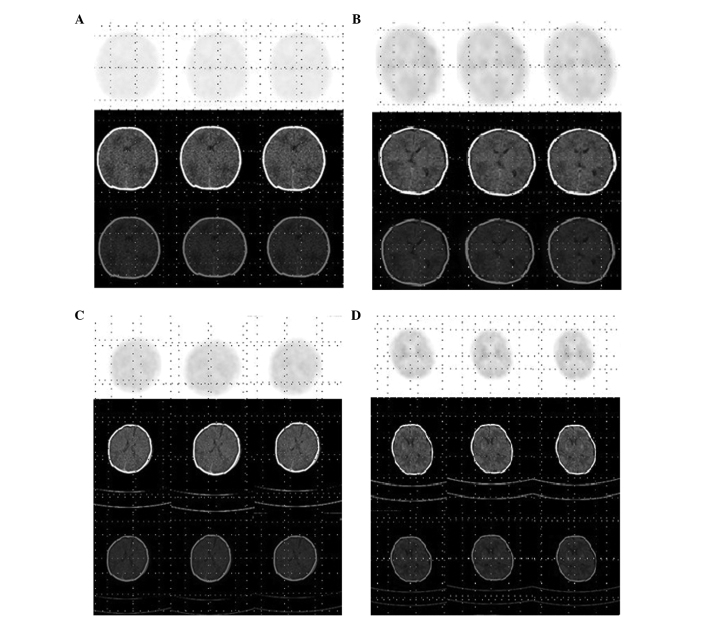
Comparisons of the rates of glucose metabolism prior to and following treatment. (A) Images prior to mild hypothermia therapy. The images show diffuse low density and low metabolism in the bilateral brain tissue. (B) Images following mild hypothermia therapy. The metabolism of the brain tissue has recovered. The brain images are sharp, clear and symmetrical. (C) Images prior to conventional therapy. The images show diffuse low density and low metabolism in the bilateral brain tissue. (D) Images following conventional therapy. The metabolism of the brain tissue has recovered significantly, but the level of recovery is lower compared with that of mild hypothermia therapy. In each figure: first row, images following positron emission tomography (PET) alone; second row, images following computed tomography (CT) and; third row, reconstructed images for PET + CT.
